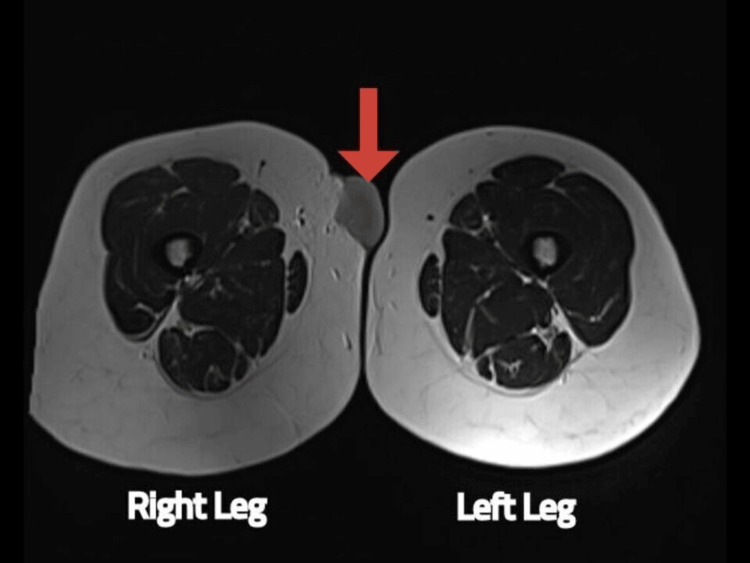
MRI of the proximal thigh shows soft-tissue swelling in the axial view MRI: magnetic resonance imaging The red arrow shows soft-tissue swelling in the right leg.
